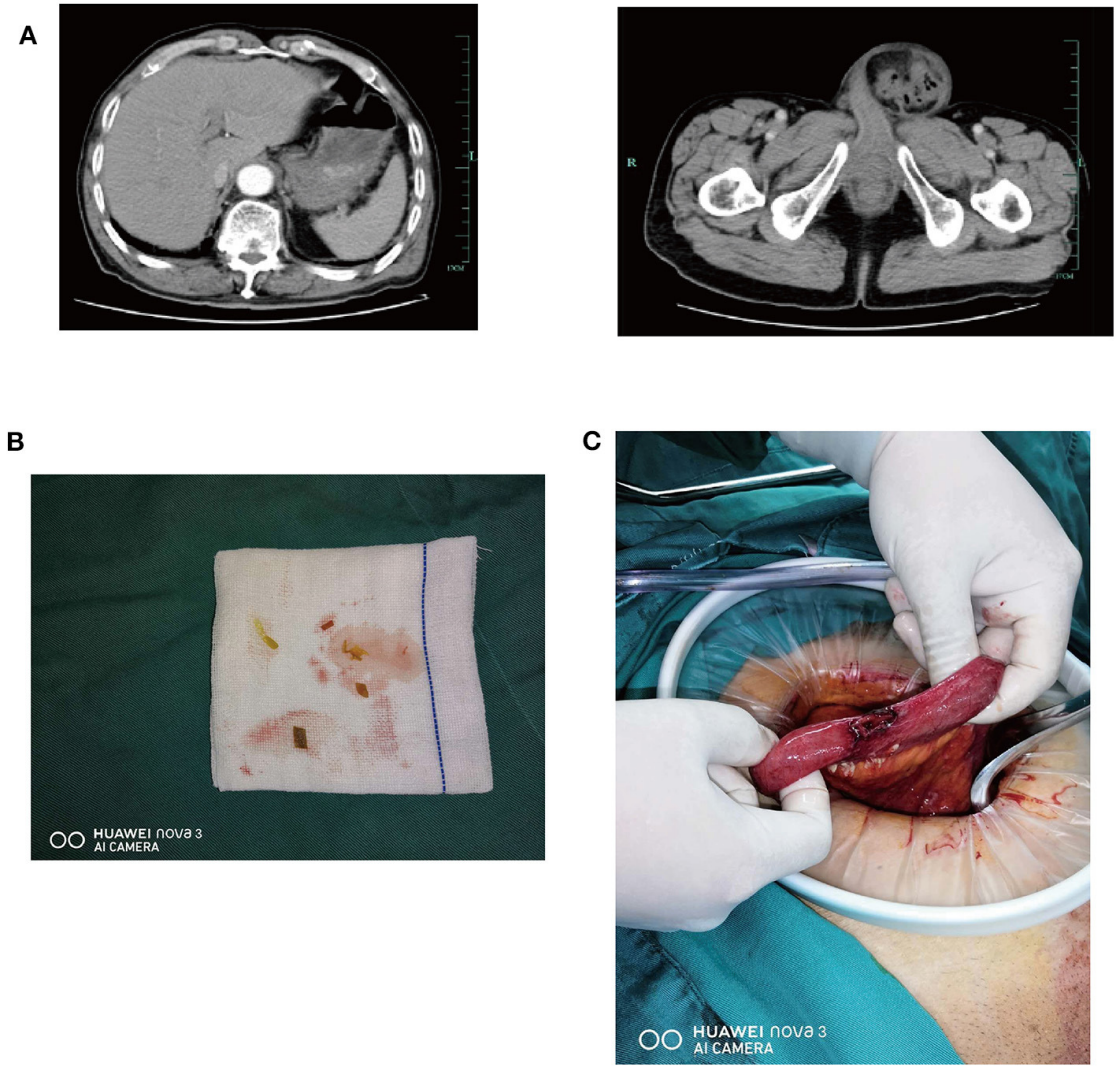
(A) Contrast enhanced CT of the abdomen in a 77-year-old male with a non-abdomen trauma. In the left CT photograph of the upper abdomen, no free abdominal gas has been found. In the right CT photograph of the pelvis, the hernia contents of the left incarcerated inguinal hernia can be seen clearly. (B) A small amount of food in ascites can be seen in the left groin which suggests the possibility of perforation of the intraperitoneal intestine. (C) In the middle part of the small intestine, there was a laceration of about 1.0 cm with obvious adhesion of purulent moss.
